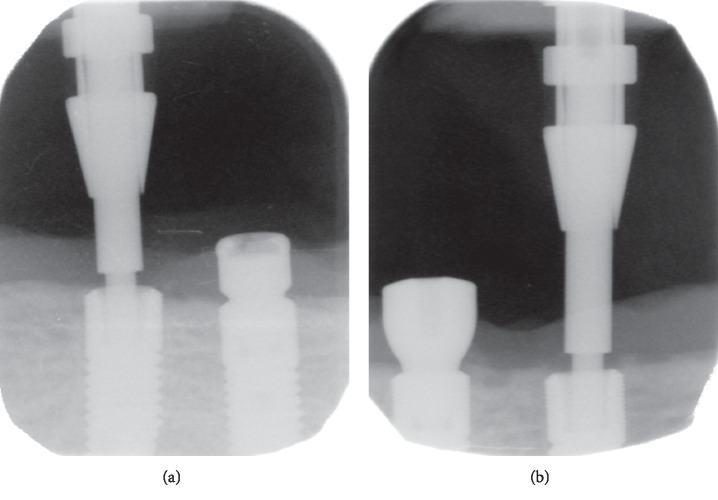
Standardized periapical radiograph taken immediately after (a) implant placement #43 and (b) implant placement #33.
